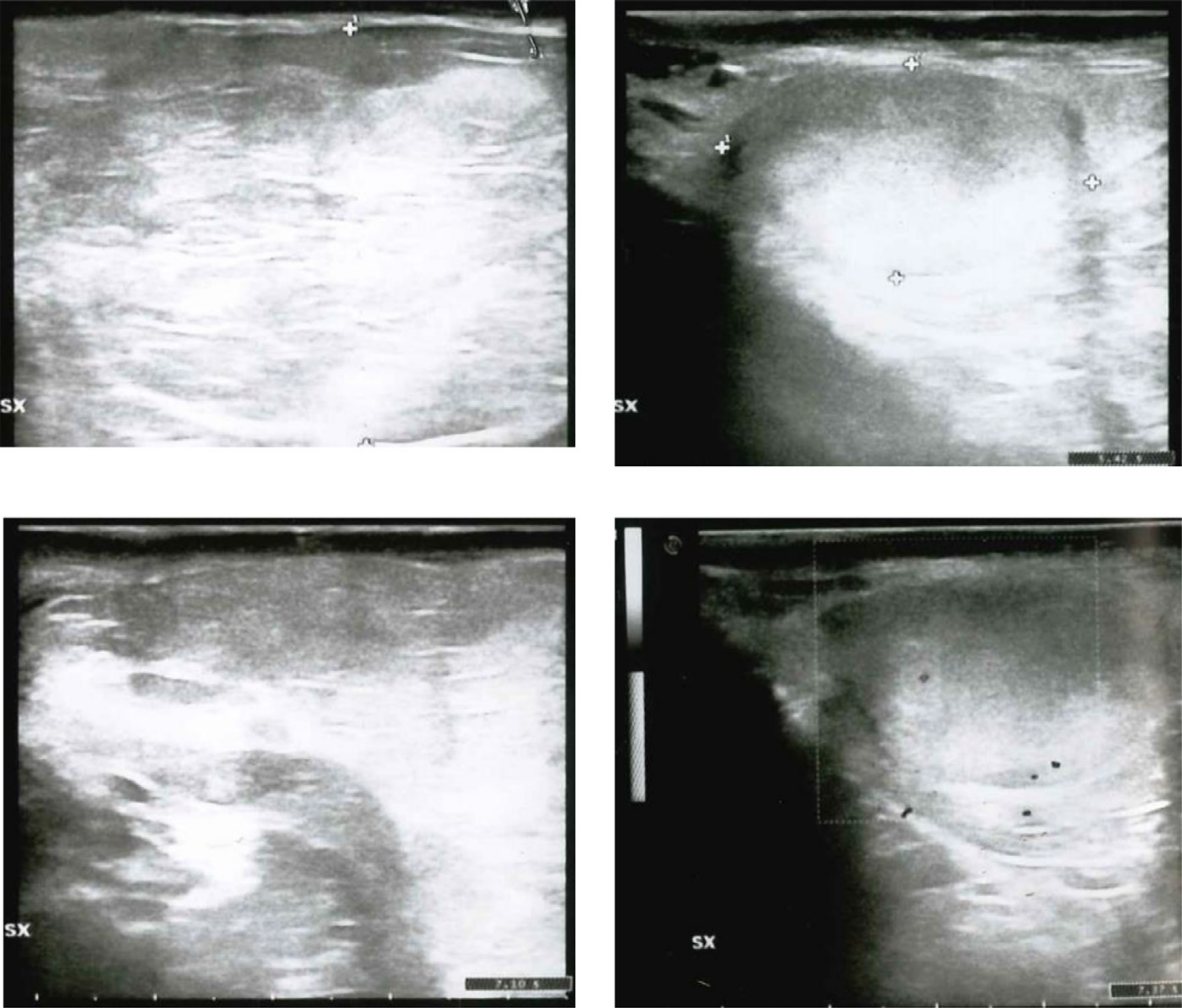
Ultrasound image of the lesion of the left spermatic cord (A and B); in homogeneously hyperechoic and poorly vascularized on Doppler (D). The above was accompanied by a left testicle of reduced size (C) compared to the controlateral (3.2 cm × 2 cm vs 4.8 cm × 2.4 cm) and with an in homogeneously hyperechoic echo structure as well as poorly vascularized (D) as from chronic tissue suffering.
